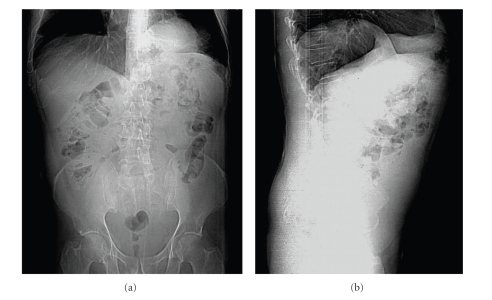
Abdominal X-ray showed a dilated loops of the colon above left hemidiaphragm.
